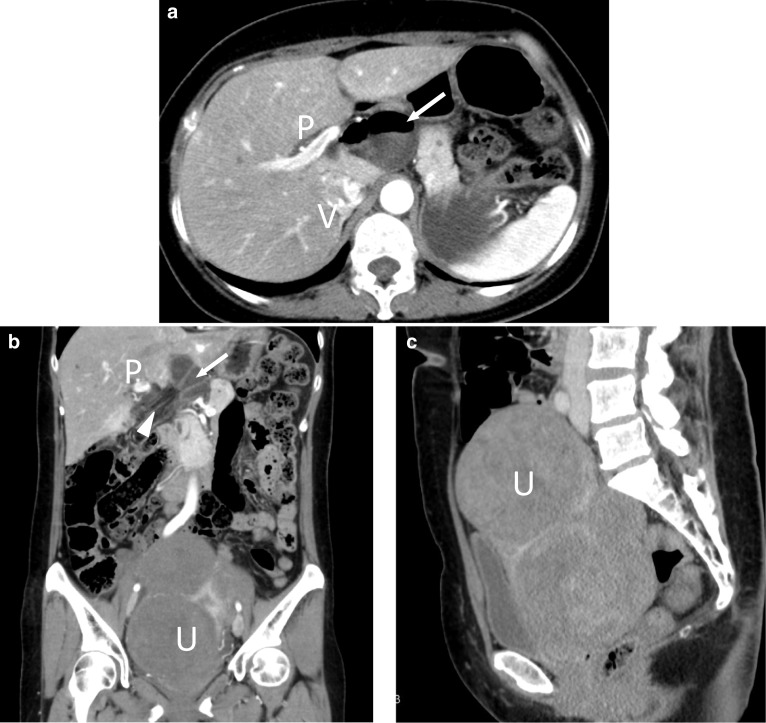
Abdominal contrast-enhanced computed tomography. a Axial image shows the dilated small bowel loop (arrow) between the portal vein (P) and the inferior vena cava (V). b Coronal image shows stretched and converging mesenteric vessels toward the foramen of Winslow (arrowheads). There are large uterine fibroids in the pelvis (U). Arrow and P indicate small bowel loops and portal vein, respectively. c Sagittal image shows the large uterine fibroids that are huge enough to occupy the pelvis (U)
